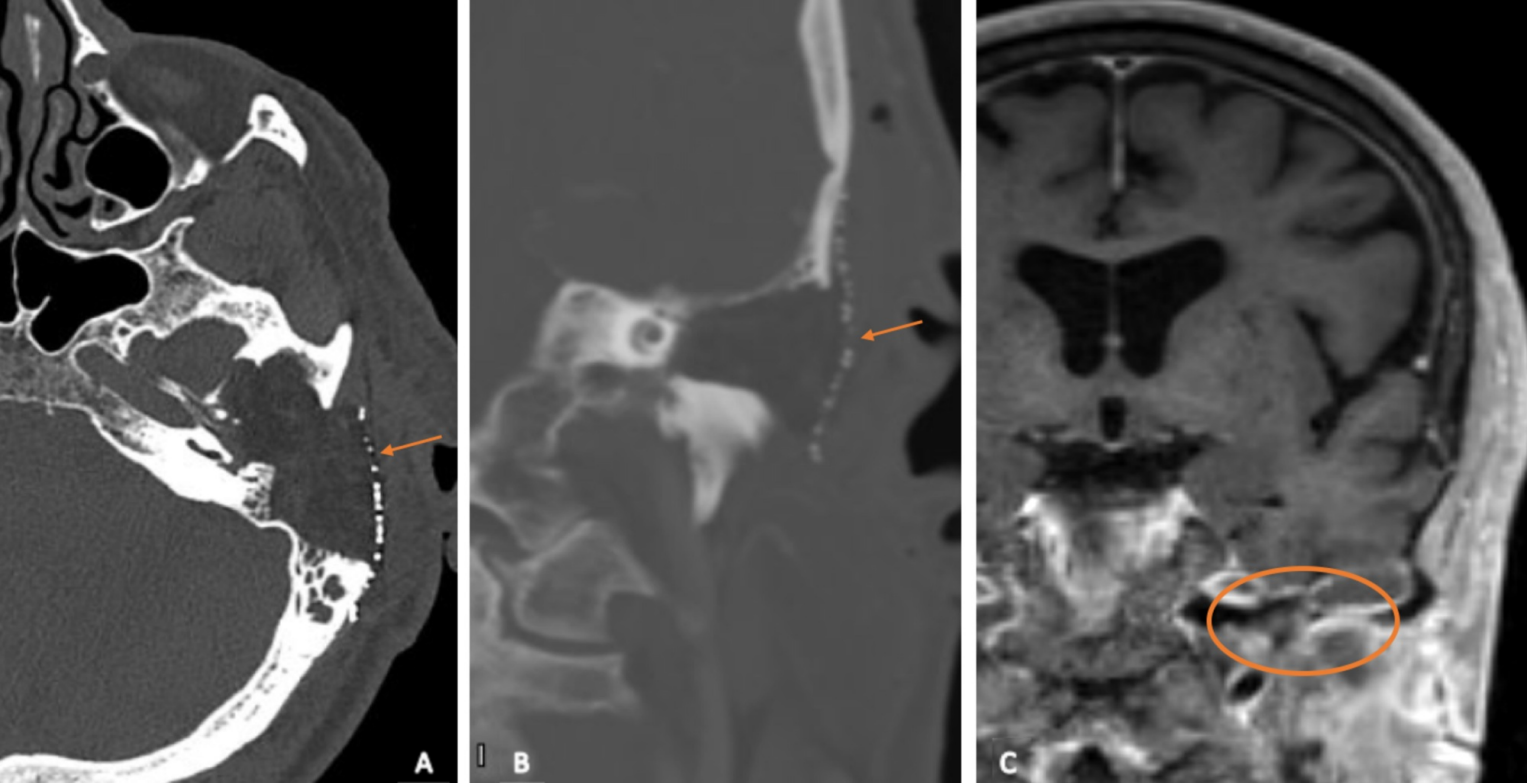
Postoperative imaging. High-resolution CT ears with contrast: (A) axial and (B) coronal view showing the subtotal petrosectomy cavity obliterated with abdominal fat and covered by a mesh plate (arrows). (C) Coronal T1-weighted gadolinium-enhanced MRI showing complete removal of the tumor (circle).
